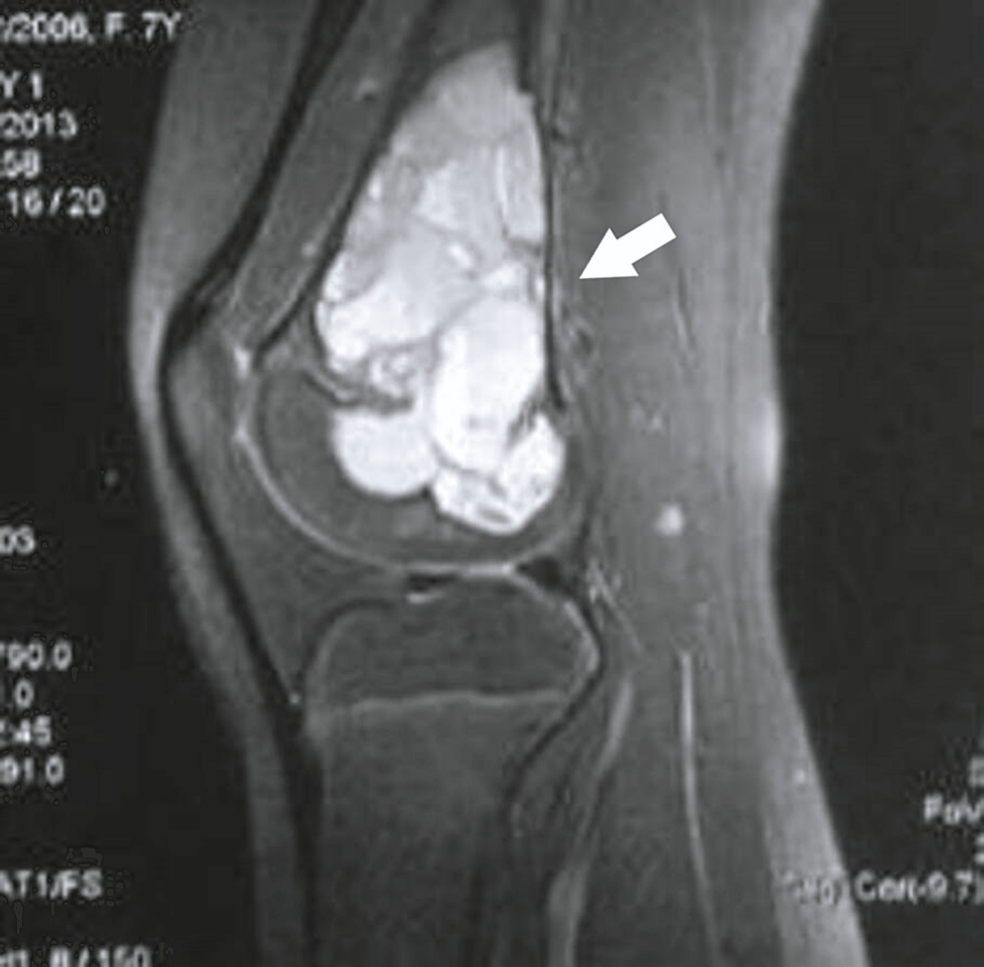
A sagittal T1 FS (Gadolinium-enhanced) weighted MRI image displaying multiple fluid-fluid levels in the metaphysis, traversing the physis and progressing into the epiphysis. Observe the thinning of the posterior cortex and the lack of a periosteal reaction. FS: Fat saturation.
